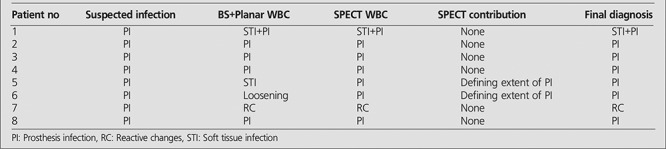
Tc 99m MDP bone scintigraphy of 22-y-old man in whom osteomyelitis of tumor prosthesis in left tibia was suspected. (a) Angiography, (b) blood pool, (c) late phase bone scintigraphy showed increased uptake in left tibia. (d) Tc 99m planar WBC scan showed faint uptake on left tibia. (e) SPECT WBC image clearly demonstrated increased uptake around tumor prosthesis. Final diagnosis was found to be prosthesis infection by surgery
